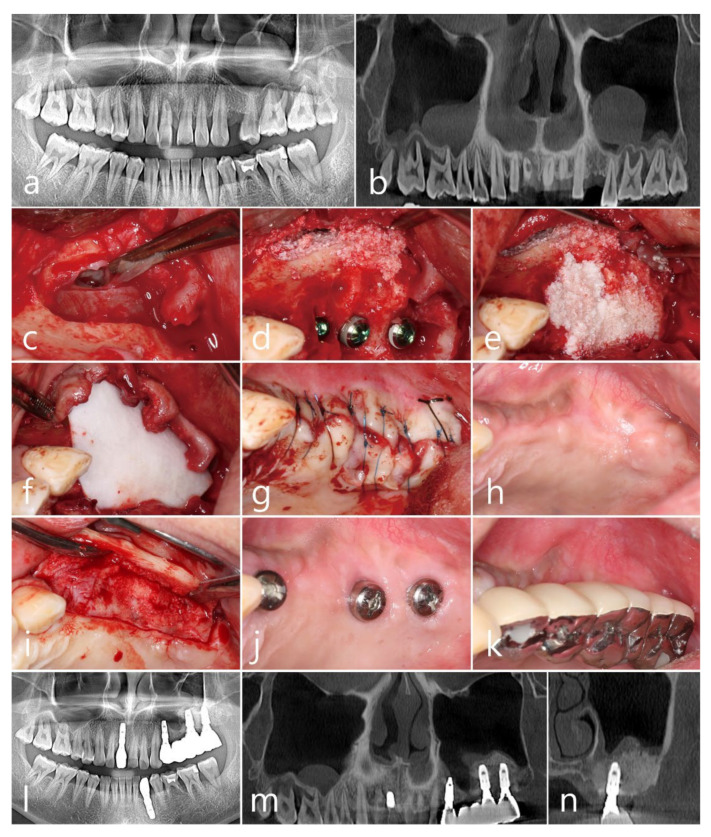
Case 4. Preoperative radiographs. Dome-shaped antral pseudocysts in both maxillary sinuses are shown in the panoramic (a) and CBCT (b) images. The “one-bony-window” technique including the preparation of an elliptical lateral window opening at the left maxilla and the removal of the antral pseudocyst through intentional perforation (c), sinus bone augmentation with implant placement (d), additional bone graft for peri-implant bone defects covered by a resorbable collagen membrane (e,f), and flap closure (g). The clinical appearance after 6 months of healing (h), at surgical reentry (i), connection of healing abutments (j), and prosthetic delivery (k). Panoramic (l) and CBCT (m,n) images at the 1-year follow-up.
